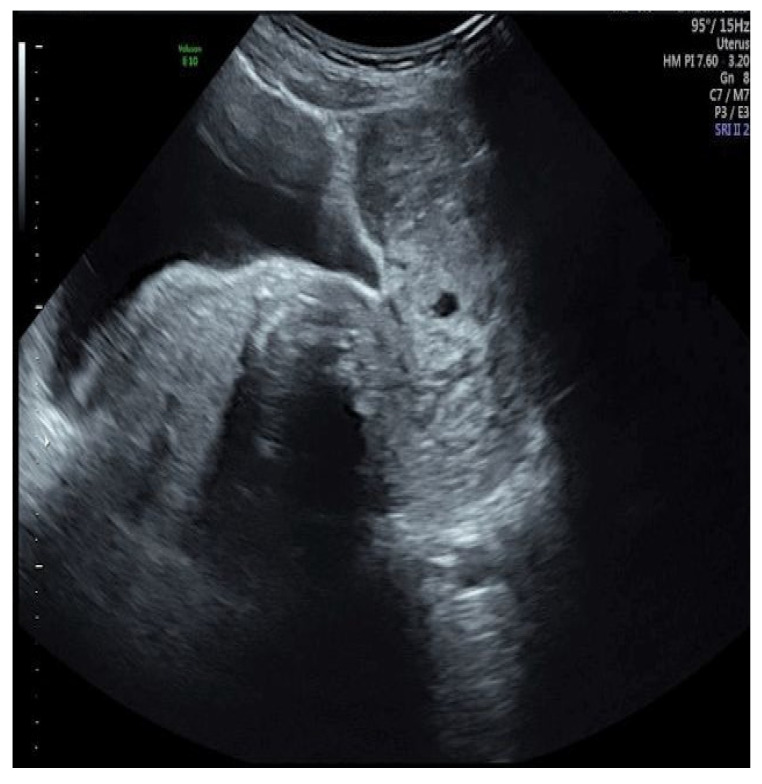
Ultrasound image of the lesion.
